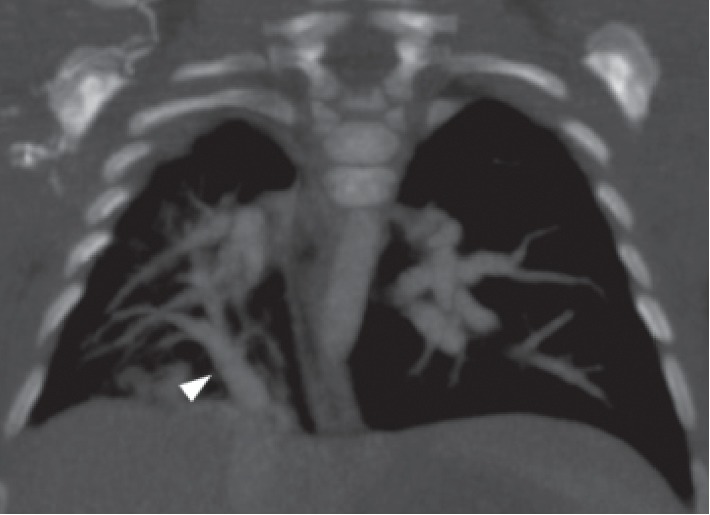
Contrast-enhanced computed tomography examination showed partial anomalous pulmonary venous drainage from the right lung into the inferior vena cava (arrow).
